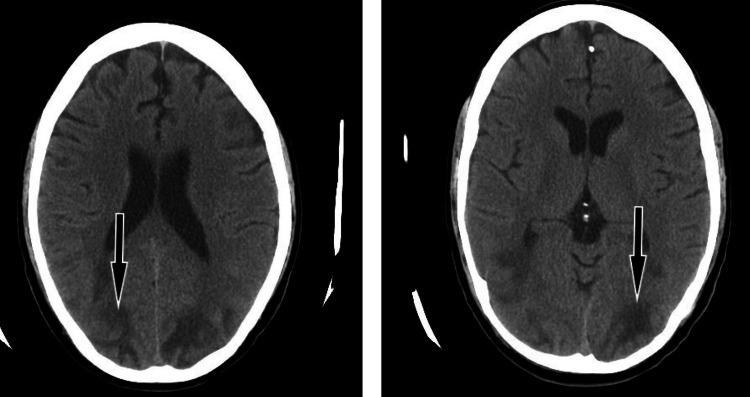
Axial non-enhanced CT scan of the brain. There are areas of ill-defined hypoattenuation involving predominantly the subcortical white matter in both parietal and occipital lobes (arrows). There is no acute intracranial hemorrhage. All images are the original work of the author.
